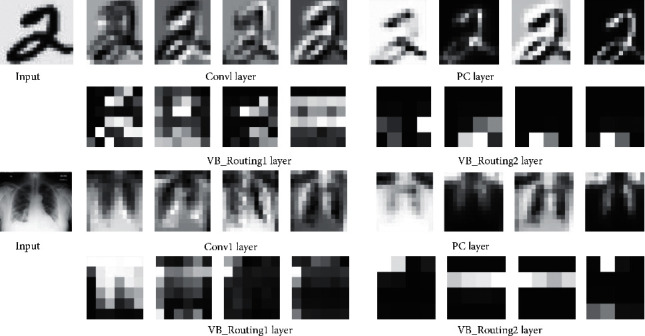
Visualization of the feature maps for the layers in the VMG-Routing model. The first two rows show the MNIST input image and the outputs of each layer. The second two rows show the input from a COVID-19 Radiography image and the corresponding outputs of the filters for each layer.
